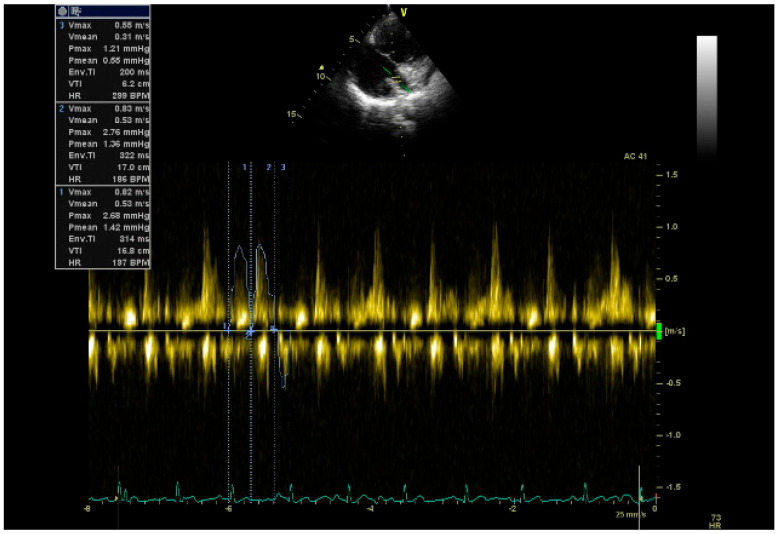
Transthoracic echocardiography-based Doppler measurements of CS flow parameters. In this modified image, PW Doppler measurements reveal a triphasic CS flow pattern: forward systolic and diastolic components, followed by a retrograde diastolic flow representing right atrial contraction. Abbreviations: coronary sinus (CS), pulsed wave (PW).
